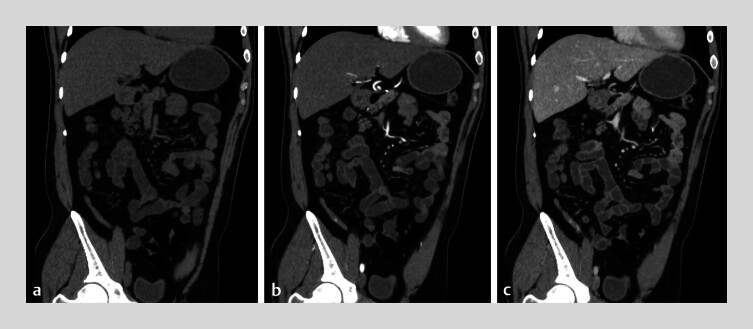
CT images of appendiceal hemorrhage.
